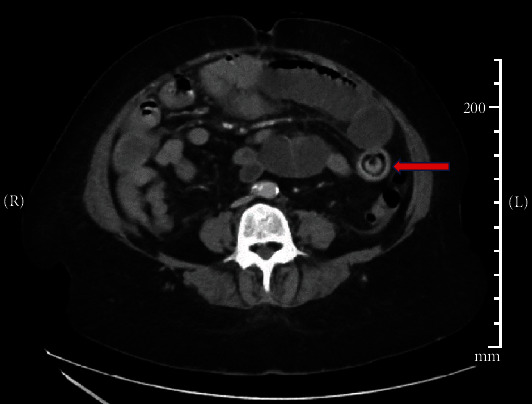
Axial CT demonstrating intraluminal mass causing SBO.
